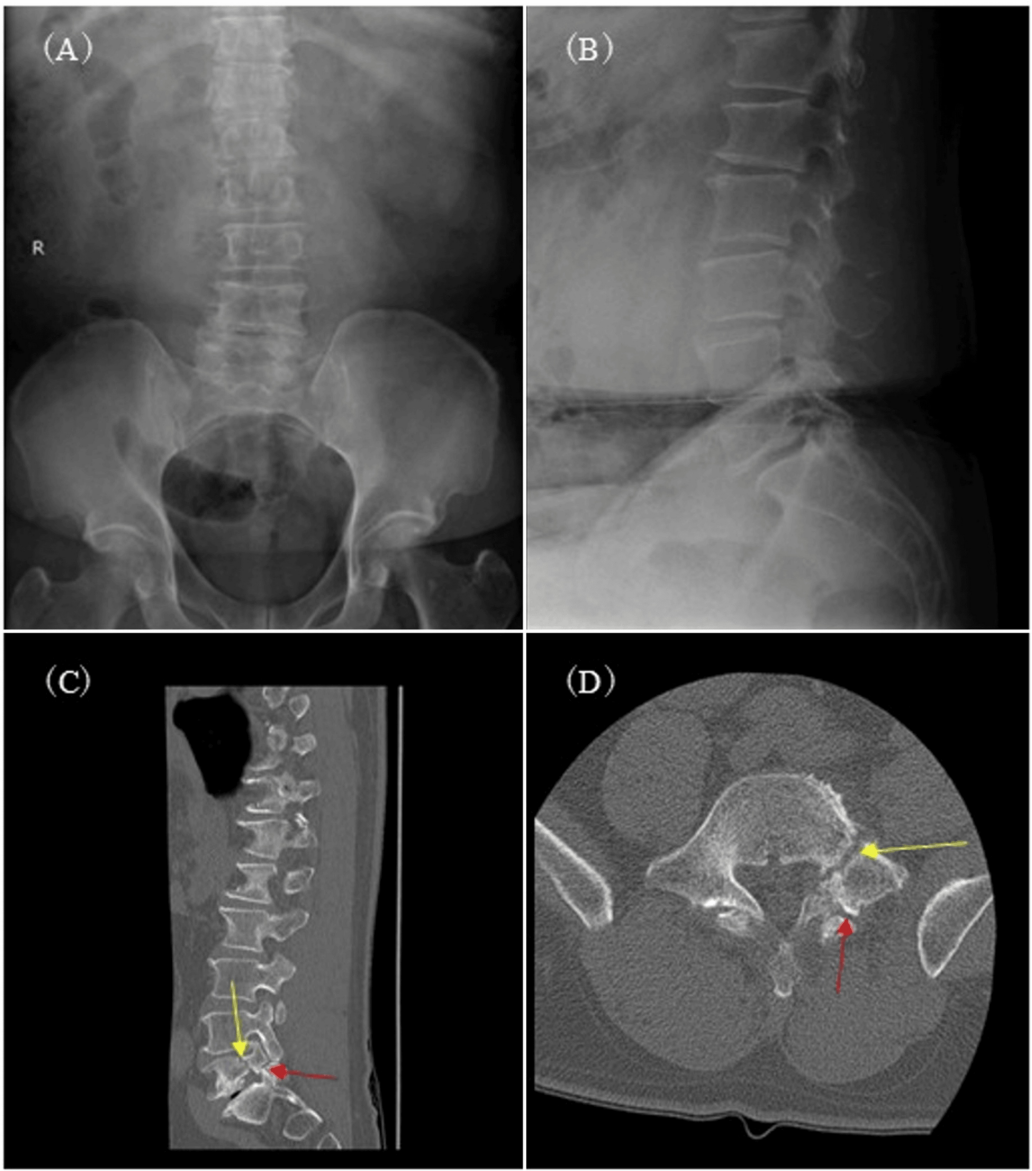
Lumbar X-ray and CT results CT: computed tomography Preoperative radiographs and CT images demonstrating L5 spondylolysis with an ipsilateral pedicle fracture. Left-sided spondylolysis (red arrows) and a concomitant ipsilateral pedicle fracture (yellow arrows) are clearly identified at the L5 level. (A) Anteroposterior radiograph, (B) lateral radiograph, (C) sagittal CT image, (D) axial CT image
